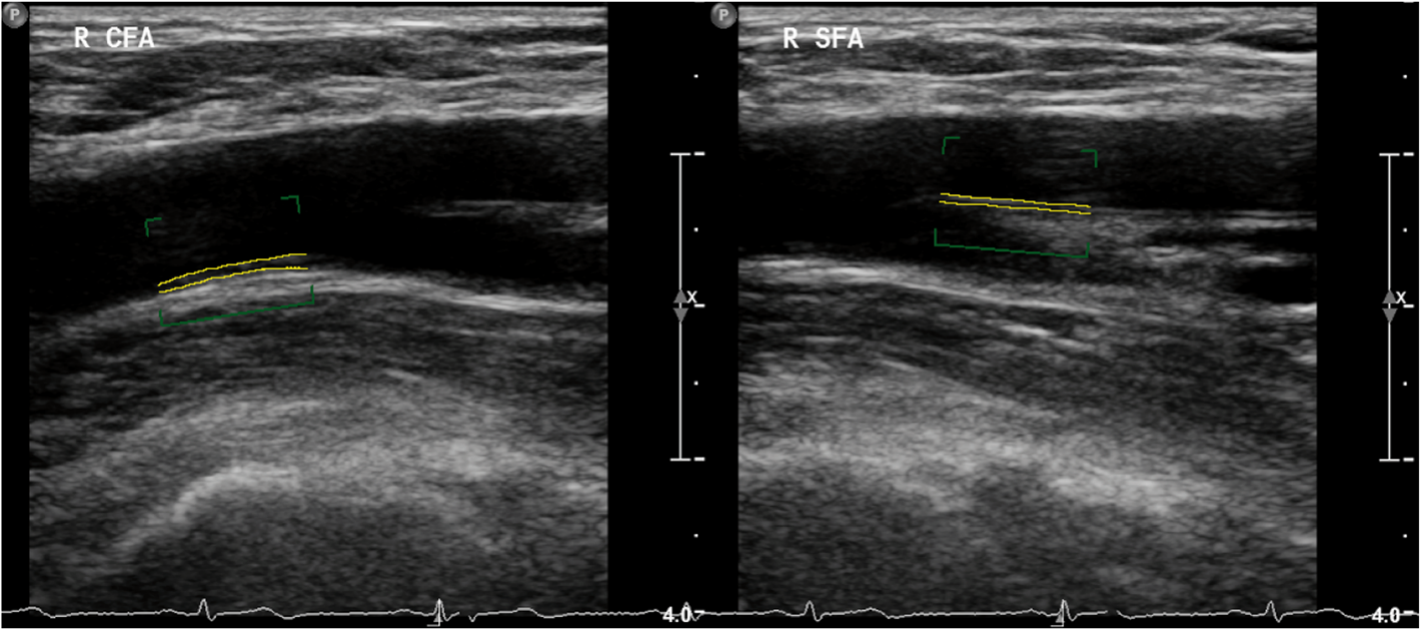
B-mode ultrasonography and IMT measurement in the distal CFA (left) and the proximal SFA segment (right) performed by QLAB software.
